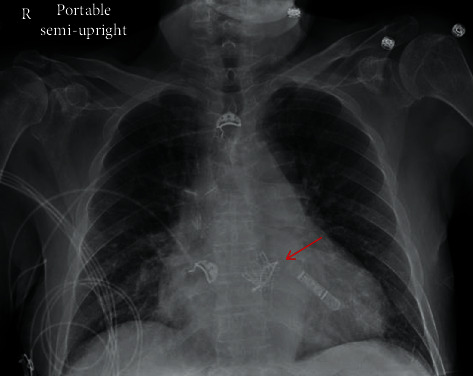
Chest X-ray (CXR): there are mildly increased interstitial markings (chronic in character), cardiomegaly, and no evidence of consolidation or pleural effusion. Loop monitor can be visualized in the CXR at the left lower chest wall as well as a valve-in-valve TAVR (red arrow).
